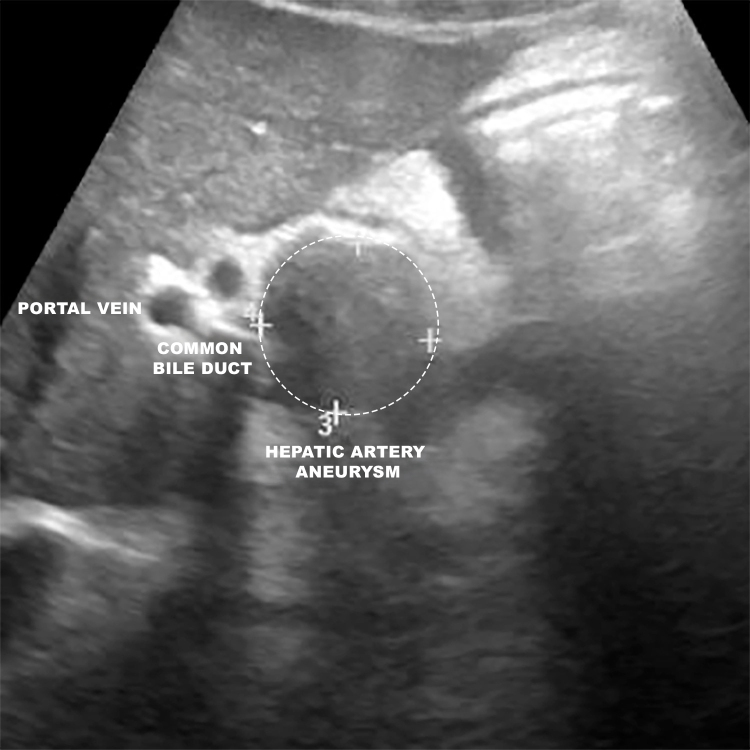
Upper abdominal ultrasound demonstrating a heterogeneous lesion, predominantly echolucent in the topography of the hepatic hilum, with well-defined margins and anatomic correlation with the portal vein, common bile duct, duodenum, and head of the pancreas.
